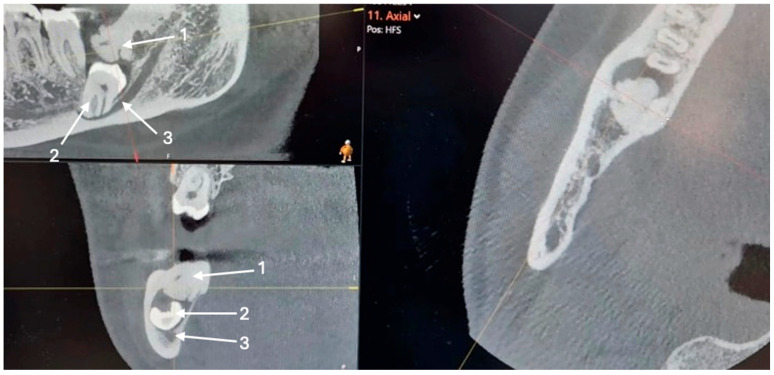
CBCT image showing the impacted tooth 48 and associated bone changes, where 1 indicates a sclerotic bone change, 2 an impacted mandibular third molar (tooth 48), and 3 the inferior alveolar nerve canal.
